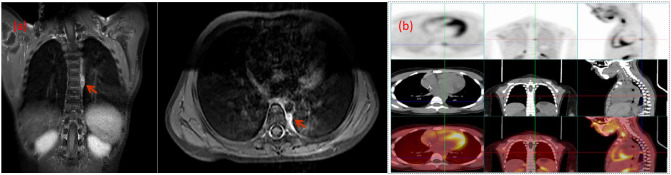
These images were acquired in July 2024 to September 2024 following the completion of consolidation and anti-GD2 immunotherapy (Dinutuximab beta). Prior to this scan, standard ^123I-MIBG scintigraphy was entirely negative. (A) Contrast-enhanced chest MRI (coronal and axial views) demonstrates persistent thickening of the paravertebral soft tissue on the left side, spanning thoracic vertebrae T3 to T9 (red arrows). (B) Given the structural remnants and the negative MIBG result, SSTR-targeted ^68Ga-DOTA-NOC PET/CT was performed to evaluate tumor viability. The scan reveals a focal nodular lesion with slightly increased SSTR expression on the left margin of the T7 vertebra (measuring approximately 0.5×0.5cm, SUVmax​=3.46).
